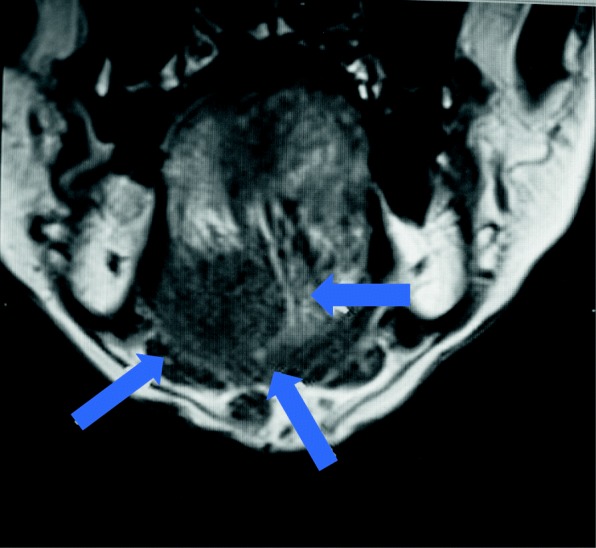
MR imaging a 70 year old male complaining of burning tongue. He was found to have a carcinoma of the left submandibular gland
